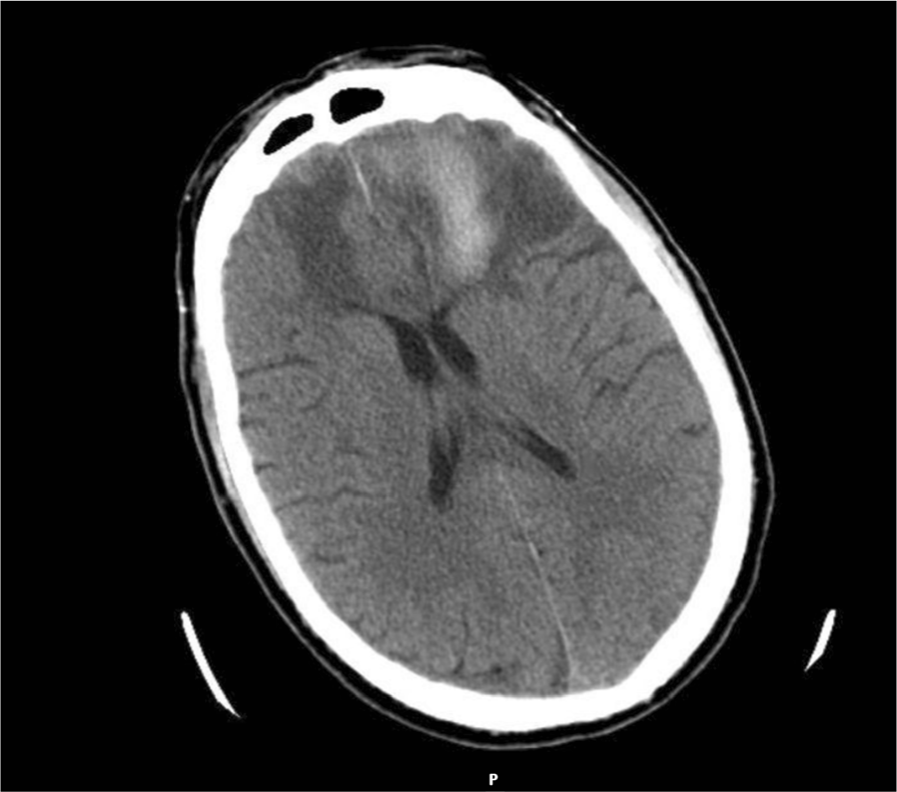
CT scan image of the brain of CASE 2 after 2 weeks of initial presentation suggesting partial resolution of the hemorrhage and no further progression or new hemorrhages coinciding with the clinical improvement.
